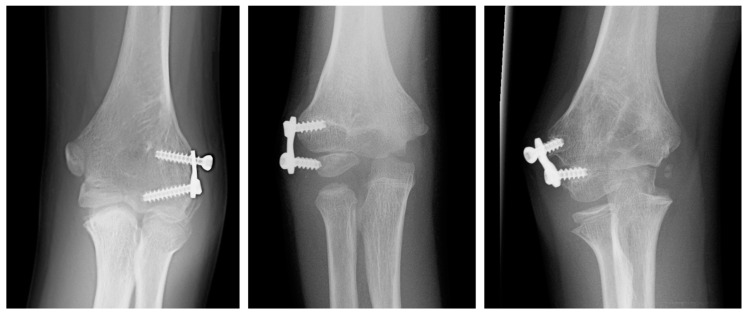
Radiographic images of some of the cases of aseptic loosening due to short screw length and a suboptimal technique in which the screws required replacement with longer and better-positioned screws.
